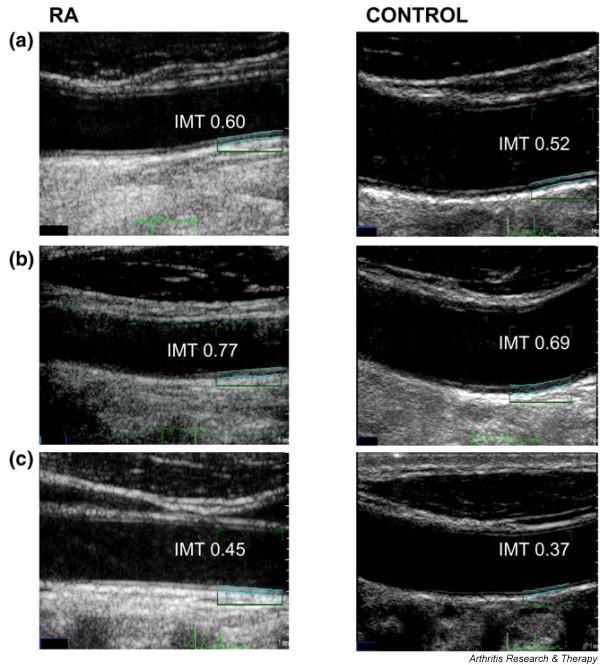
Carotid intima media thickness in rheumatoid arthritis patients compared with matched healthy control individuals. The carotid intima media thickness (IMT) is increased in rheumatoid arthritis (RA) patients compared with healthy control individuals matched for age, sex and cardiovascular risk factors. Representative carotid ultrasound images from three matched RA patient and control individual pairs: (a) a 42-year-old male with RA and a 40-year-old control male, (b) a 58-year-old male with RA and a 58-year-old control male, and (c) a 31-year-old female with RA and a 31-year-old control female. The automated measurement lines over the region of interest are boxed (in blue).
